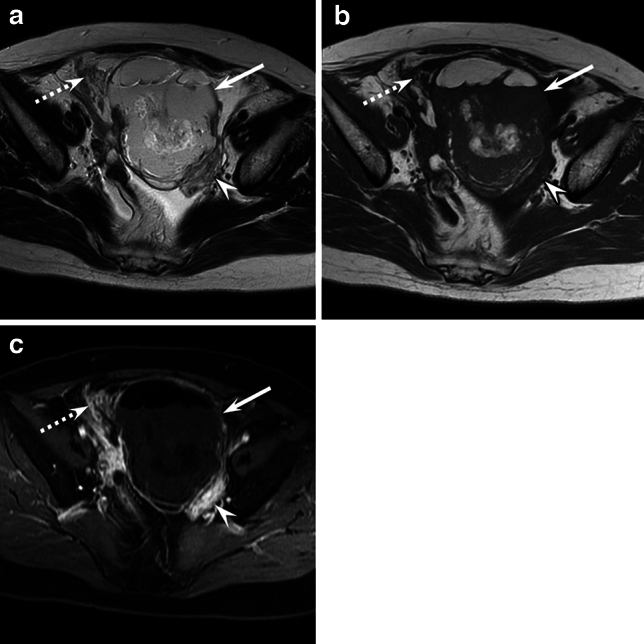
A 55-year-old woman with MT-MCT (stage IIIC). Axial T2-weighted image (a), T1-weighted image (b), and axial fat-suppressed contrast-enhanced T1-weighted image (c) showing an ill-defined fat-containing mass (arrow) with exophytic sessile solid components (arrowhead) and peritoneal dissemination (dotted arrow). The occupancy rate of fat components accounting for 23%
